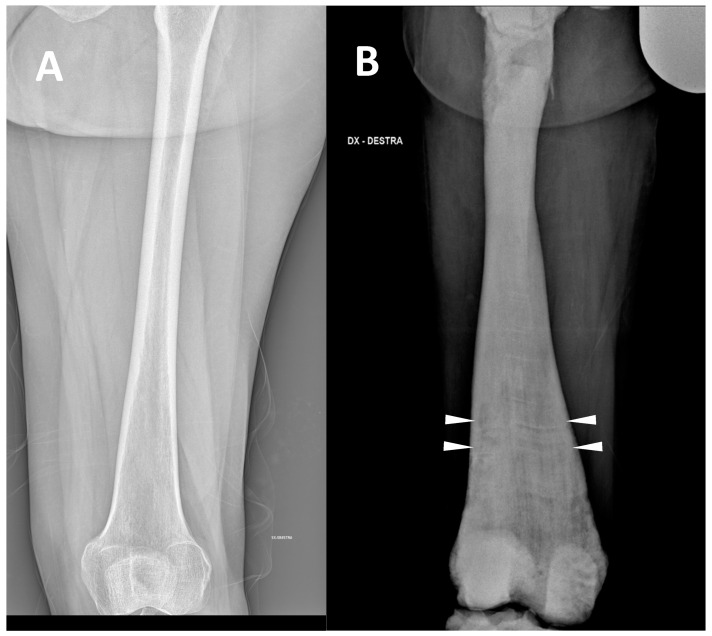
(A) conventional radiography of the thigh of a young healthy female (shown as comparison). (B) conventional radiography of the right thigh in a 35-year-old male affected by osteopetrosis (ARO type). Metaphyseal enlargement with the so-called ‘Erlenmeyer flask deformity’ of the distal femur, as well as a diffuse increase in bone radiolucency (increased bone density) are detectable. Alternating lucent and sclerotic metaphyseal banding can also be noted.
